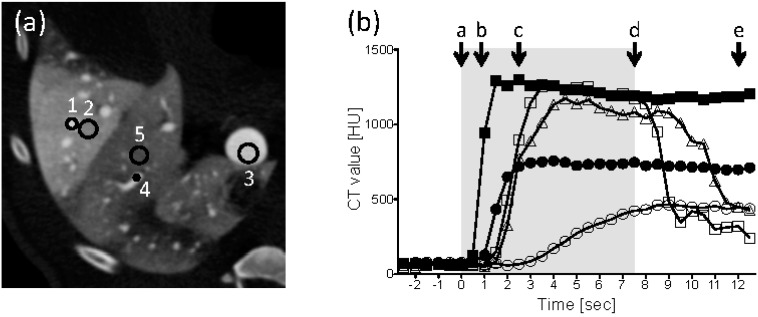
Tissue intensity during a regional hydrodynamic injection. (a) The image from Figure 2d is shown with a different window width and center to represent the vessels. The regions of interest that were used to measure the CT values were the HV (target), liver parenchyma (target), the main trunk of the PV, a peripheral PV branch (non-target), and liver parenchyma (non-target), which were marked 1 to 5, respectively. (b) Time intensity curves. A period of the injection is indicated by a gray box. The time points corresponding to the Figure 2a–e are represented by a–e. Closed squares: HV (target); closed circles: parenchyma (target); open squares: main trunk of the PV; open triangles: peripheral PV (non-target); open circles: parenchyma (non-target).
